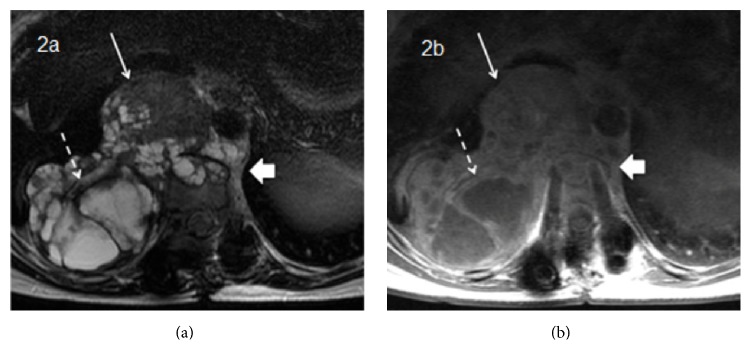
Baseline (pretreatment) MRI findings on T2-weighted (a) and postgadolinium T1-weighted (b) images showing a mass arising from the vertebral body (wide arrow), with a heterogeneous soft tissue component with solid (narrow arrow) and cystic areas (dashed arrow).
